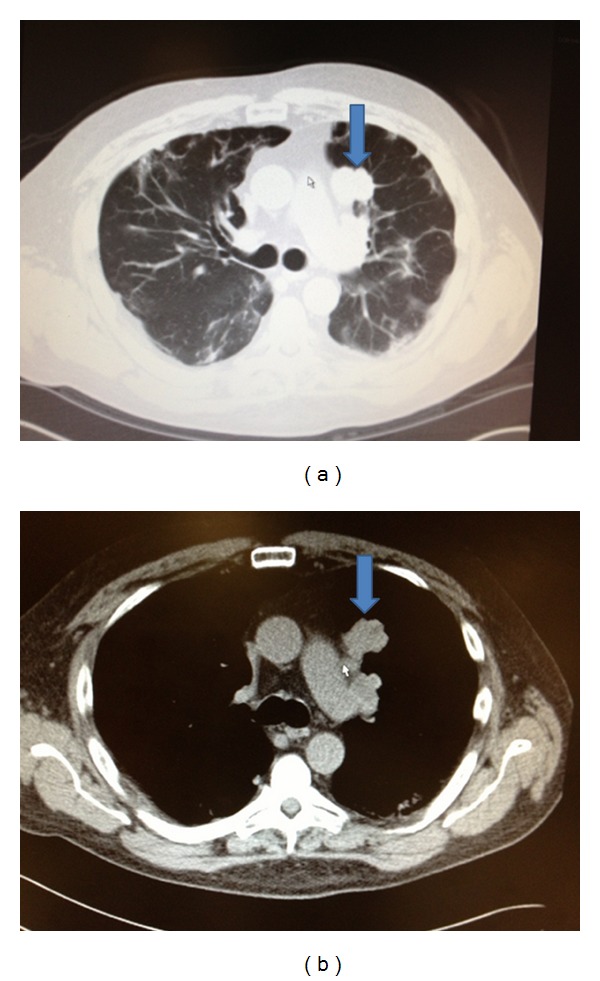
Chest CT with contrast that showed RCC metastasis to the left lung (blue arrow) in lung and mediastinum window.
